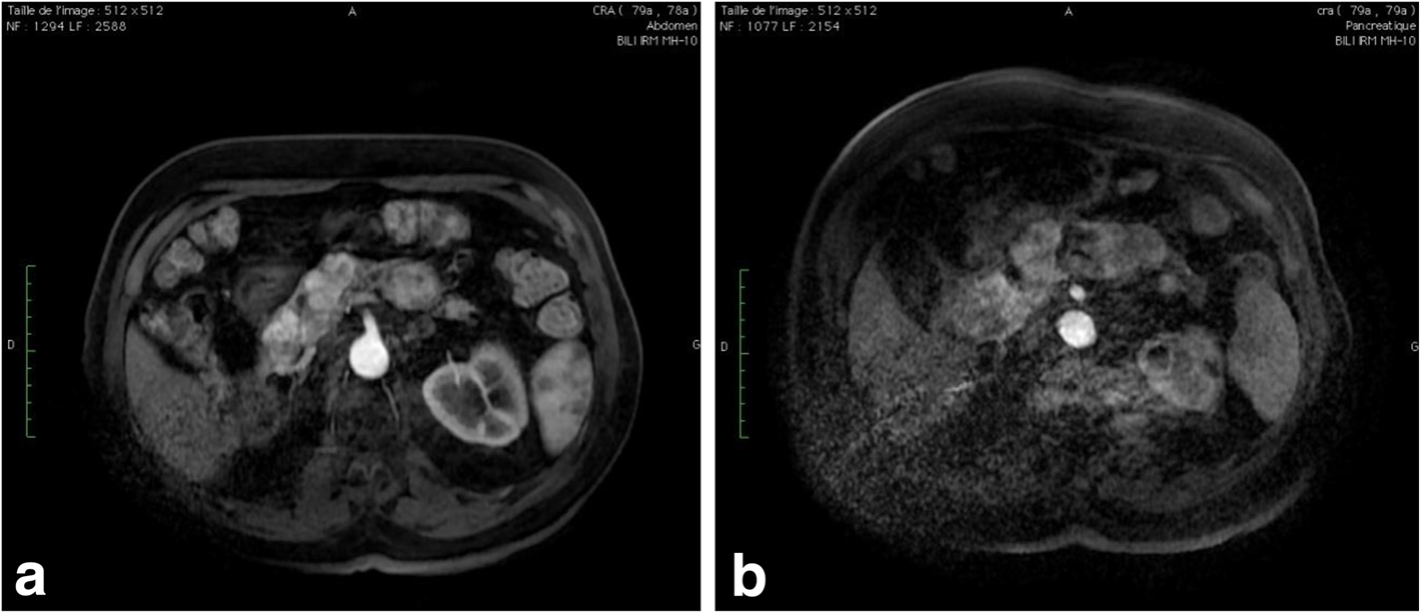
Magnetic resonance imaging shows the exemplary course of pancreatic metastases from renal cell cancer. The magnetic resonance imaging at the *left* side was performed directly before the treatment with a tyrosine kinase inhibitor was stopped in July 2016 (a), the MRI at the *right* side 13 months later in August 2017 (b).
